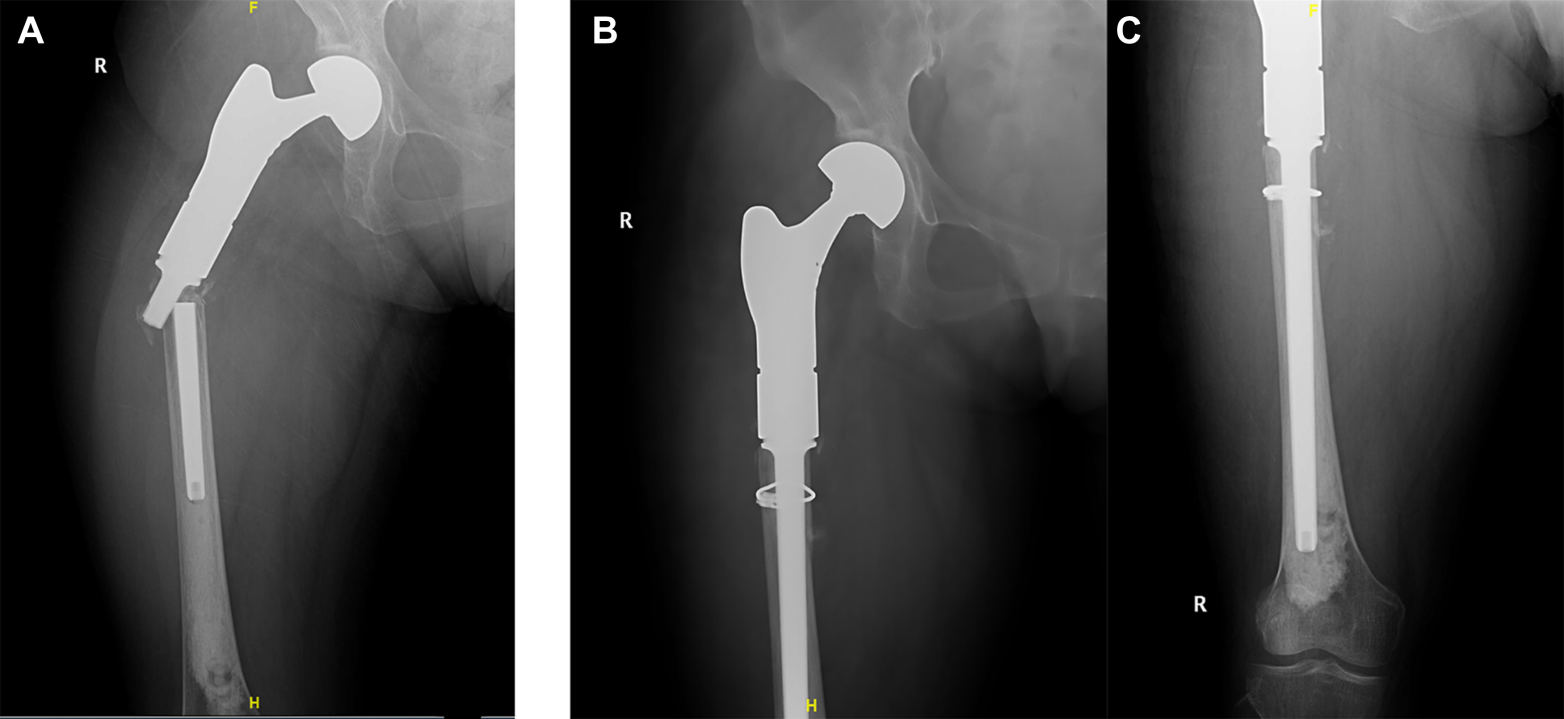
(A) The x-ray film showed breakage of femoral stem at 12 months postoperatively; (B,C) revision surgery was performed and prosthesis was replaced by a new and longer femoral stem.
